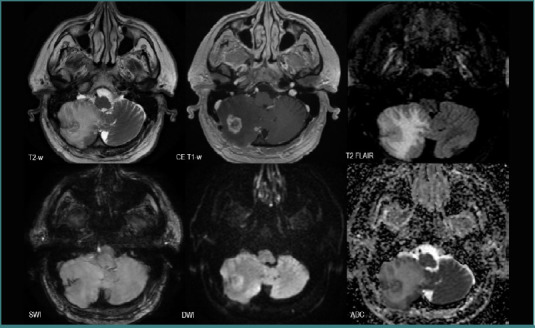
MRI brain acquisition of large cell carcinoma BMs. Highly edematous right cerebellar lesion (high T2-w and T2-w FLAIR signals of the surrounding white matter), with intralesional vascularization (SWI), central necrosis, mild peripheric diffusion (high DWI and low ADC), and contrast intake (CE T1-w).
